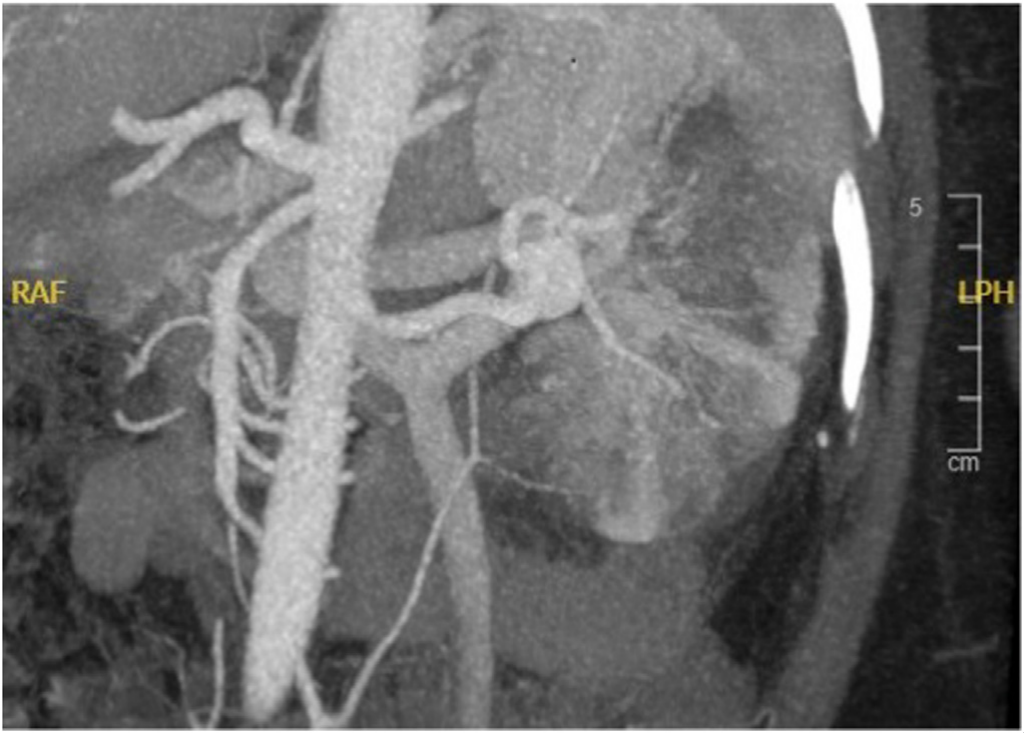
Computed tomography angiogram showing distal left renal artery aneurysm (RAA) involving superior and inferior segmental arteries and renal infarct involving mid- and lower pole of kidney.
